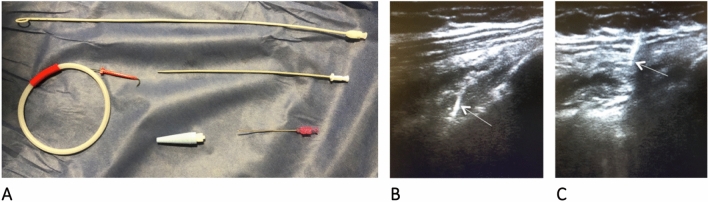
A Thoracentesis kit B. Evaluation with a 13-MHz linear transducer at the level of the fifth to seventh intercostal space along the mid-axillary line, verifying the absence of pleural sliding at the puncture site; once vessel interposition is ruled out with color Doppler, the puncture is made; the passage of the needle to the pleural space is visualized in real time; visualization is lost upon entry into the pleural space given the presence of air (arrow) C. A guide wire is passed, verifying entry into the pleural space (arrow); as with the needle, visualization is lost when entering the pleural space
